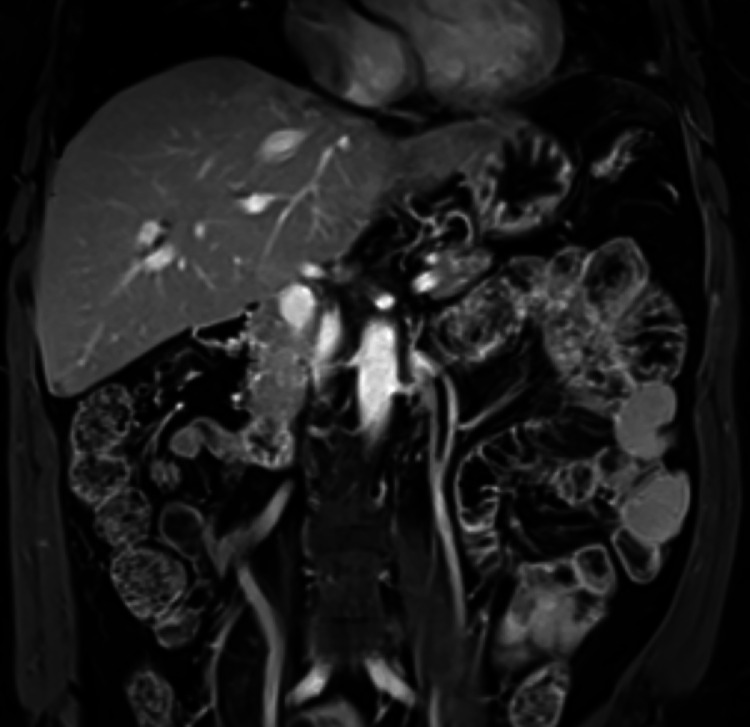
Most recent follow-up T1-weighted post-contrast MRI obtained 16 months after en-bloc resection demonstrated complete response without evidence of residual or recurrent disease.
